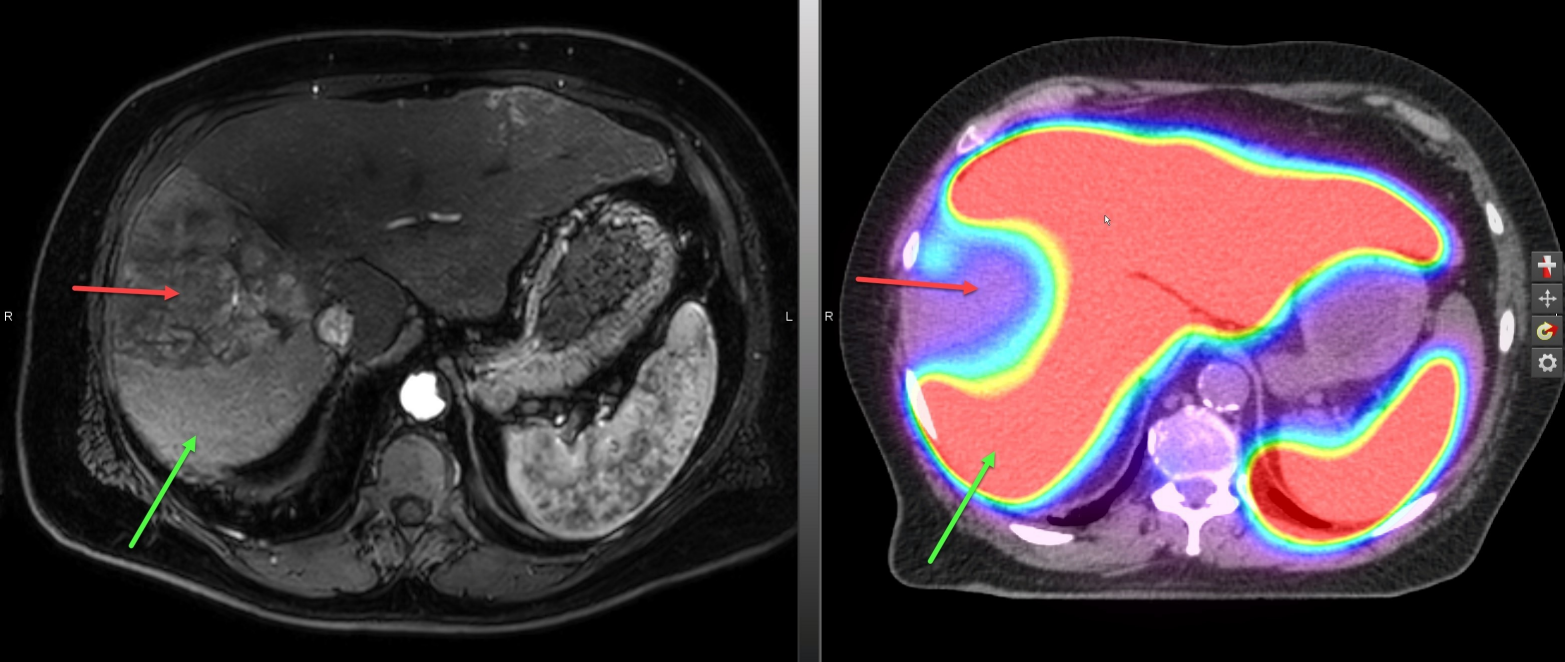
The treatment planning sulfur colloid single-photon emission computed tomography (SPECT/CT) scan. The technetium-99m [^99m^Tc] sulfur colloid SPECT/CT scan with which images sulfur colloid uptake in liver Kupffer cells and has been shown to correlate with global clinical liver function, was used to help identify and delineate both gross tumor and uninvolved normal liver. Areas of high sulfur colloid uptake represent non-GTV liver (red color wash) and the large well-defined photopenic defect (red arrow) corresponds to the tumor. The presumed perfusion abnormalities seen on the magnetic resonance imaging (MRI) scan (left panel) show high uptake of sulfur colloid on SPECT/CT (green arrows) and provides confirmation that these areas in the liver are unlikely infiltrated with tumor (red arrow).
